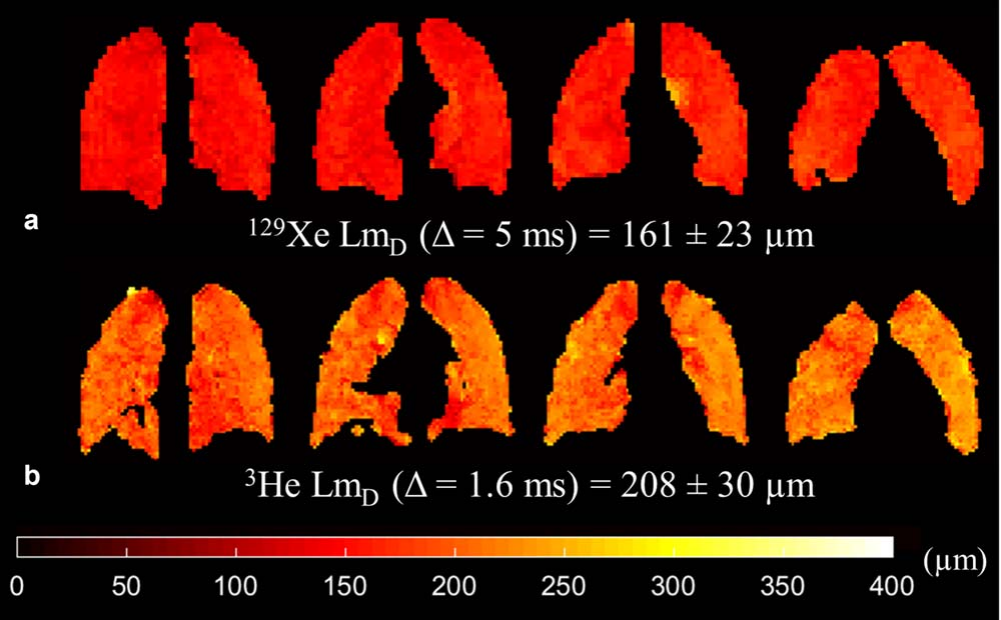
Prospective CS results for a healthy volunteer (HV1) (SNR = 30). (a) Example ^129^Xe Lm_D_ maps derived from 3D multiple b‐value ^129^Xe DW‐MRI. (b) Example ^3^He Lm_D_ maps in comparative slices demonstrate the mismatch in Lm_D_ values between the two nuclei.
